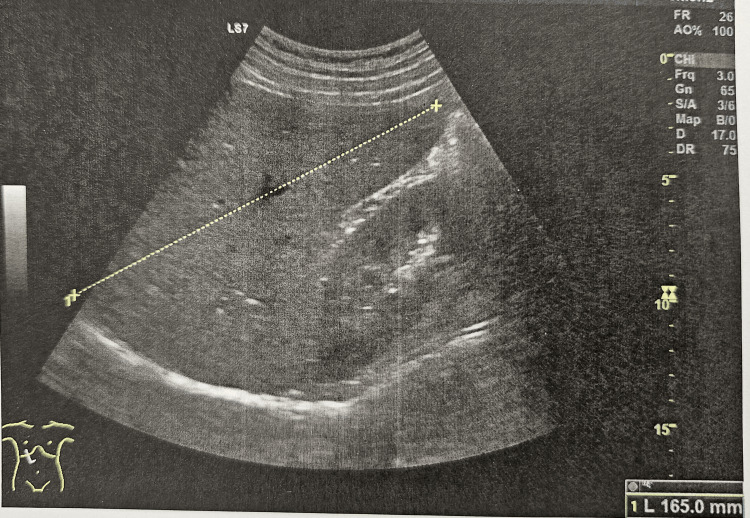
Ultrasound image of hepatomegaly.
